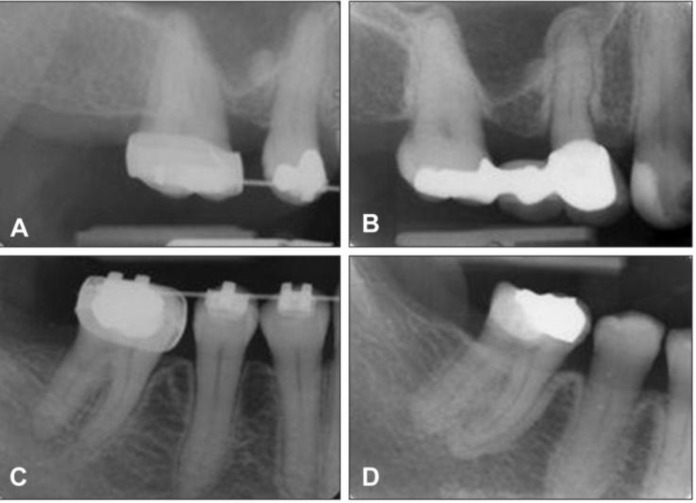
Periapical radiographs of a woman, showing generalized osseous sclerosis ‎of uniform thickness involving the cortical plate and lamina dura. A and C radiographs shows the ‎pre-bisphosphonate therapy condition of the cortical plates and the lamina dura. B and D ‎radiographs shows thickening of the cortical plate and lamina dura after 2 years bisphosphonate ‎therapy. No clinical signs and symptoms were established at this initial stage
